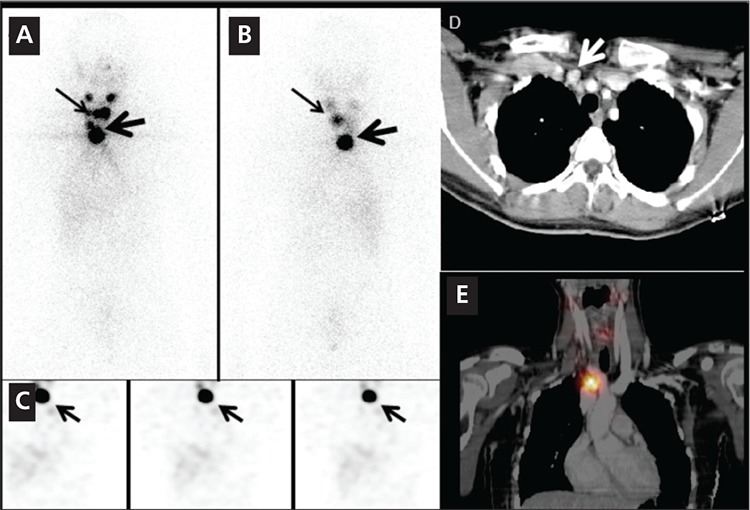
A 15 year-old girl with papillary thyroid cancer and totalthyroidectomy underwent radioiodine therapy with 3700 MBq I-131. The1 week post-therapy anterior (A) and posterior (B) whole body imagesshowed bilateral intense tracer uptake in the region of the submandibularglands and focal intense uptake in the thyroid resection bed (thin arrows).A suspicious focus of intense increased tracer uptake is noted in the lowerneck/upper mediastinum, (thick arrows, A,B). Selected coronal SPECTimages (C) of the neck obtained on the same day show focal intense uptakein the upper mediastinum (arrows, C). SPECT alone does not localize theuptake. Axial CT image (D) shows an enlarged 1.3x1.2 cm right upperparamediastinal lymph node (white arrow). Coronal fused image (E) showsabnormal increased tracer uptake in the enlarged lymph node seen on CT.This lymph node was correctly characterized and reported as metastaticwith SPECT/CT findings. Surgical resection of the lymph node revealedmetastatic papillary thyroid cancer
